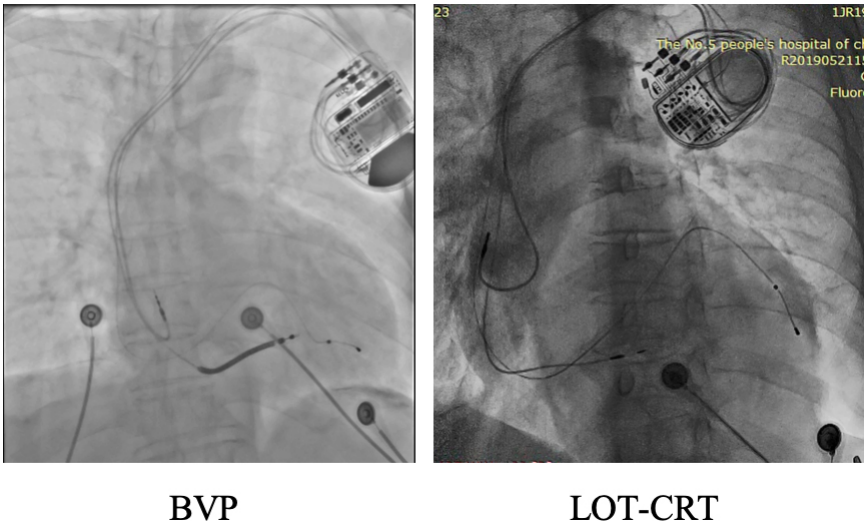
Representative chest X-rays from the first postoperative day are
